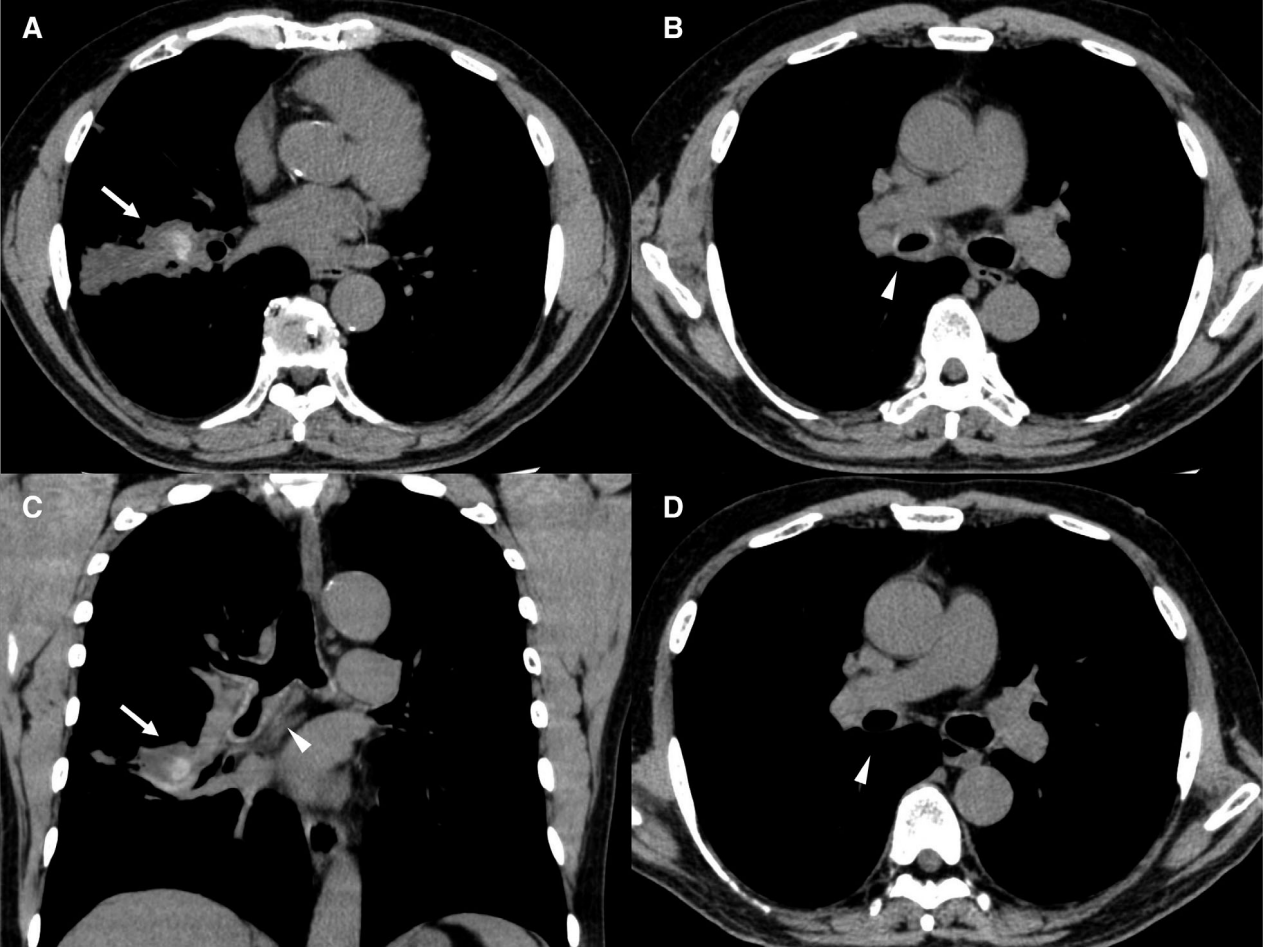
(A) Chest computed tomography (CT) findings (soft tissue window) showing highly attenuated, mucus‐filled, dilated bronchi in the right lower lobes (arrow). (B) Chest CT findings (soft tissue window) showing narrowing of the right main bronchus to middle truncus (arrowhead). (C) Coronal image of chest CT findings (soft tissue window) showing narrowing of the right main bronchus to middle truncus (arrowhead) and high‐attenuation mucus in the right B^8^ bronchus (arrow). (D) Chest CT findings (soft tissue window) after two months of treatment with systemic corticosteroid and antifungal agents showing resolution of the right tracheobronchial lesions (arrowhead). Prednisolone was reduced to 20 mg/day and the dose of voriconazole was 200 mg/day.
